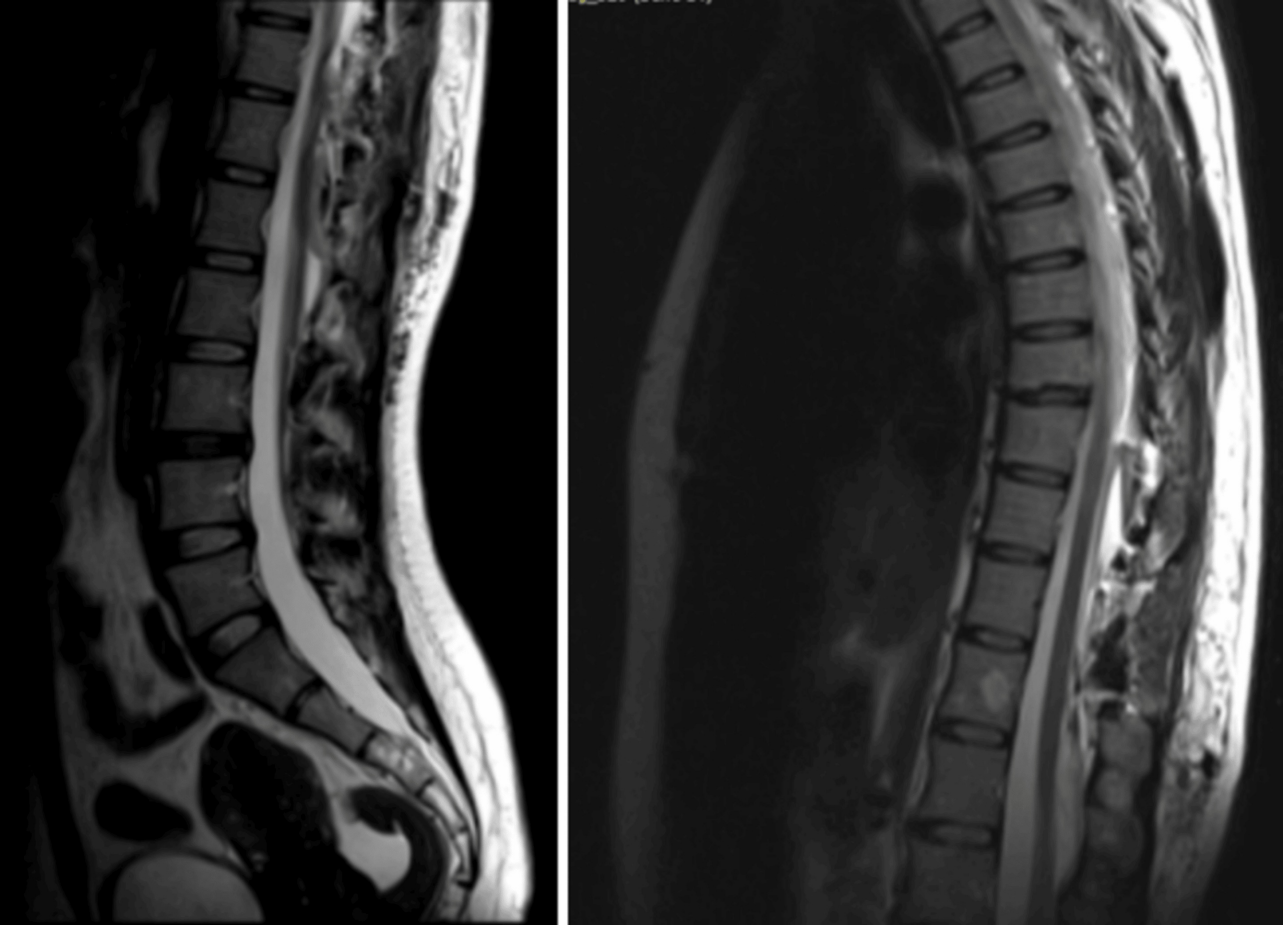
Postoperative sagittal T2-weighted MRI of the thoracolumbar spine showing resolution of the previously visualized extradural cyst and decompression of the spinal cord Postsurgical changes are evident at the T10–T12 levels.
